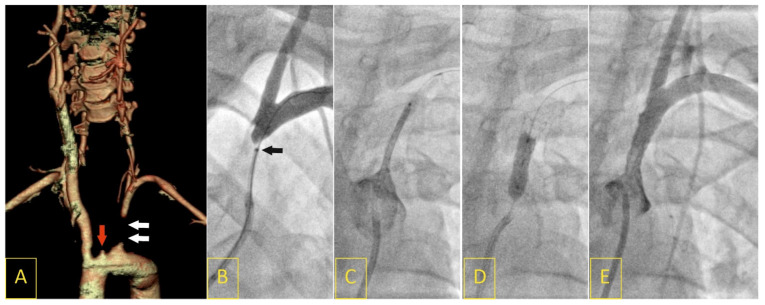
A 17-year-old female presented with “stuttering” symptoms of left-hemisphere ischemia (8 points on the National Institute of Health Stroke Scale upon admission). Computed tomography angiography showed a proximal left subclavian artery (LSA) occlusion (white arrows) with coexisting ostial left internal carotid artery occlusion (red arrow, (A)). The LSA occlusion segment was crossed with a V-18 Control Wire with a support of a NaviCross 4F catheter (tip of the support catheter marked with a black arrow (B)). Following predilatation, a drug-eluting balloon-mounted stent (Nefro 8 × 30) was implanted and postdilated (C,D). Angiography showed an optimal result of the procedure, with normal flow of the vertebral artery (E).
